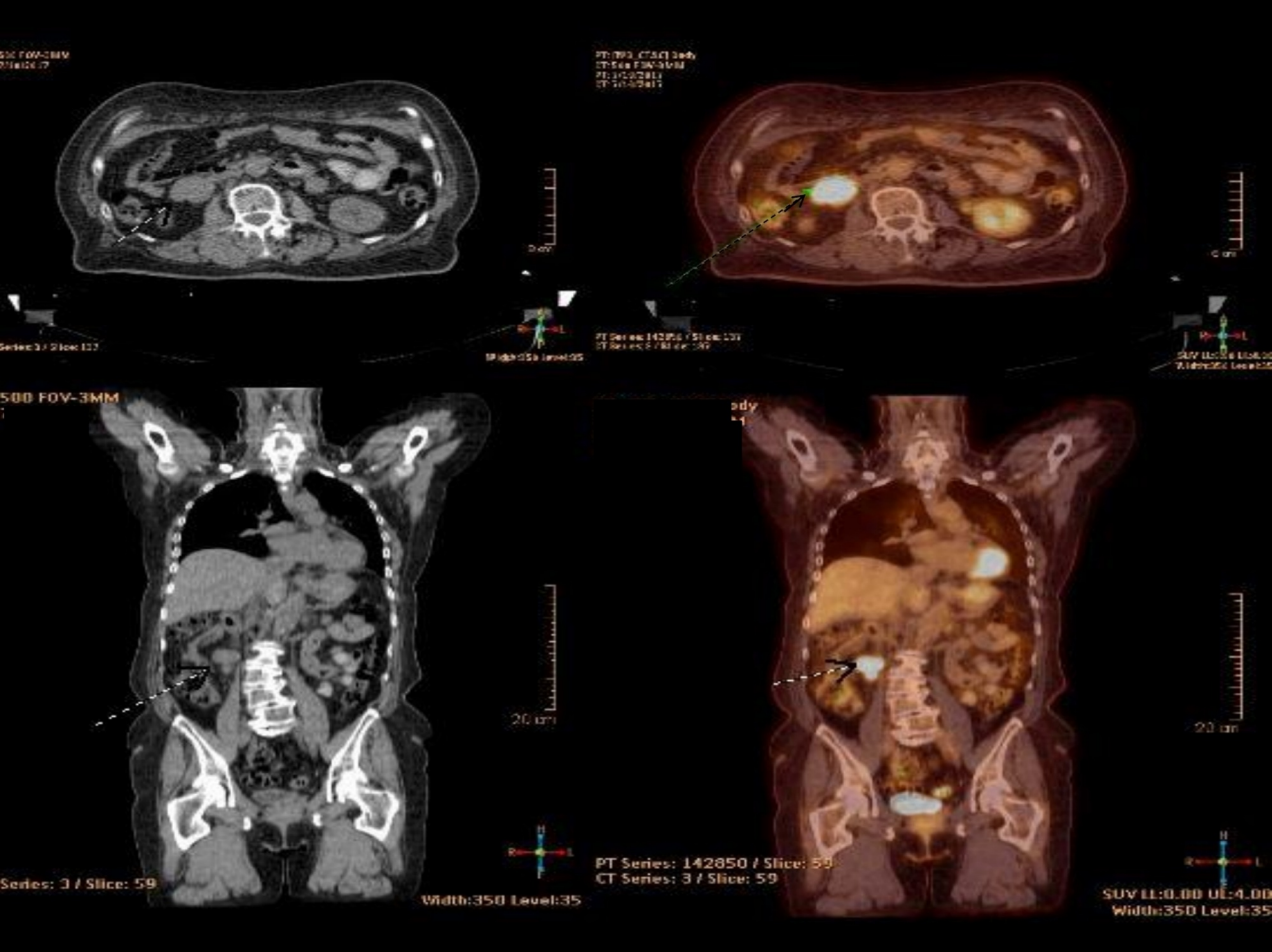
Baseline PET-CT showing the right retroperitoneal mass. PET-CT: Positron emission tomography-computed tomography
